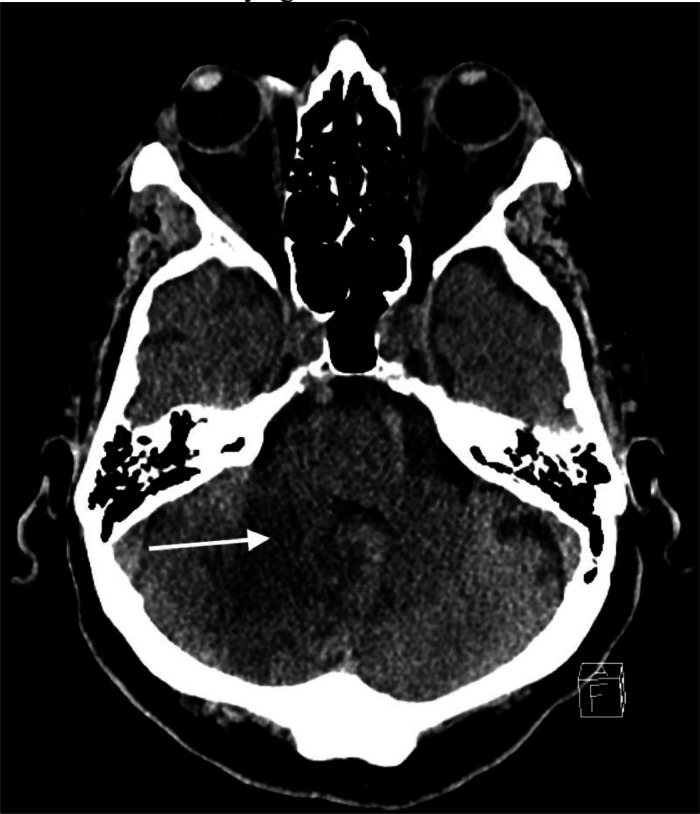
Head CT performed 2 months later, when the patient presented to our institution, demonstrated large area of hypodensity (arrow) within the right cerebellum with associated edema. The patient had originally been diagnosed with a stroke, but the persistent edema raised concern for an underlying lesion.
